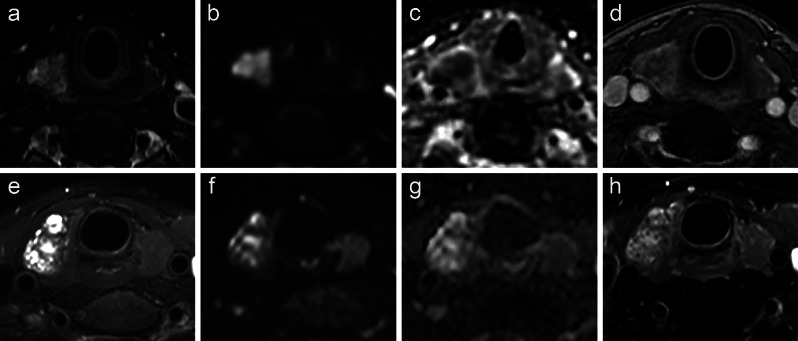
A 35-year-old female with a 1.8 cm papillary thyroid carcinoma in the right lobe of the thyroid gland (a, b, c and d) and A 47-year-old female with a 2 cm nodular goiter in the right lobe of the thyroid gland (e, f, g and h). Axial T2-weighted imaging (a) shows a striped low signal area; Axial diffusion-weighted imaging (DWI) (b) and apparent diffusion coefficient (ADC) map (c) show restricted diffusion of the lesion (high signal intensity on DWI, low signal intensity on ADC); The delayed phase contrast-enhanced T1-weighted imaging (d) reveals a greater enhancement in the peripheral area compared to the central area of the lesion, with a blurred border between them, which corresponds to the reversed halo sign in delay phase. The characteristics of the lesion (a, b, c, and d) including age < 48, low signal intensity on T2WI, restricted diffusion, and the presence of the reversed halo sign in delay phase, are consistent with an MRI risk scoring system score of 26 and indicate a determination of malignancy. Axial T2-weighted imaging (e) show a striped low signal area; Axial DWI (f) and ADC map (g) show high signal intensity of lesion; The delayed phase contrast-enhanced T1-weighted imaging (h) shows heterogeneous enhancement of lesion. The characteristics of the lesion (e, f, g and h) including age < 48 and low signal intensity on T2WI, are consistent with an MRI risk scoring system score of 6 and indicate a determination of benignity
